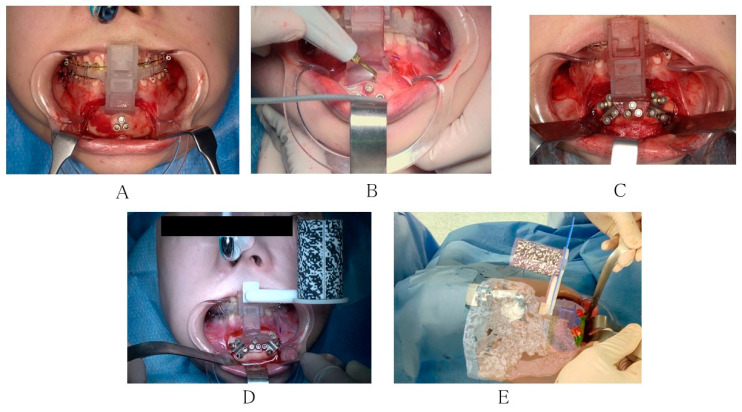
Intraoperative photo: (A) using the osteotomy device; (B) osteotomy using an ultrasonic cutting instrument; (C) repositioning device and prefixed plates attached; (D) repositioning device and registration marker connected to the splint; and (E) projecting the hologram through HoloLens 2.
